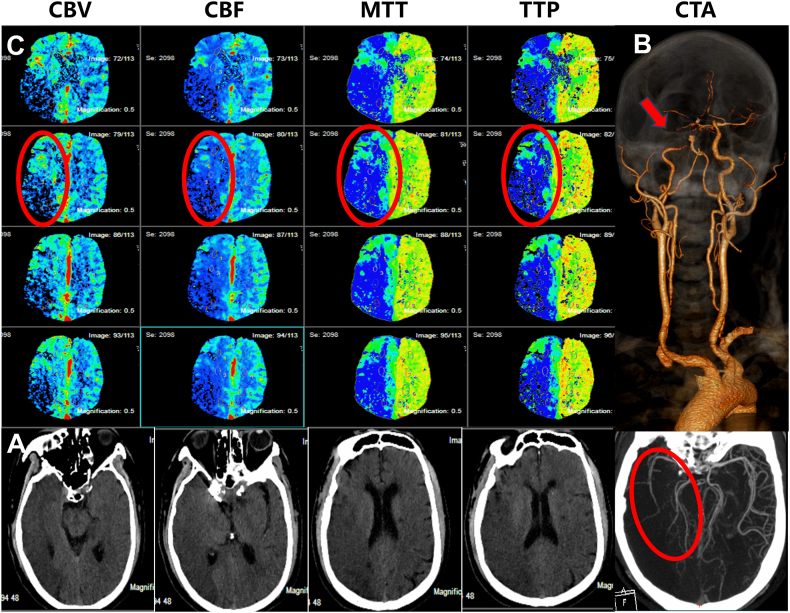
Preoperative one-stop CT examination. A: No hemorrhage was seen in the head CT scan. B: Head and neck CTA shows severe stenosis of the siphon segment of the right ICA (indicated by the yellow arrow) and sparse branching of the middle cerebral artery (indicated by the red arrow). C: Head CTP shows a large CBV area and CBF decline in the right cerebral hemisphere, extensive MTT and TTP prolongation, indicating the existence of a large-area cerebral infarction (shown in red circle). CBV cerebral blood volume; CBF-cerebral blood flow; MTT - mean transit time; TTP - time to peak. (For interpretation of the references to colour in this figure legend, the reader is referred to the Web version of this article.)
